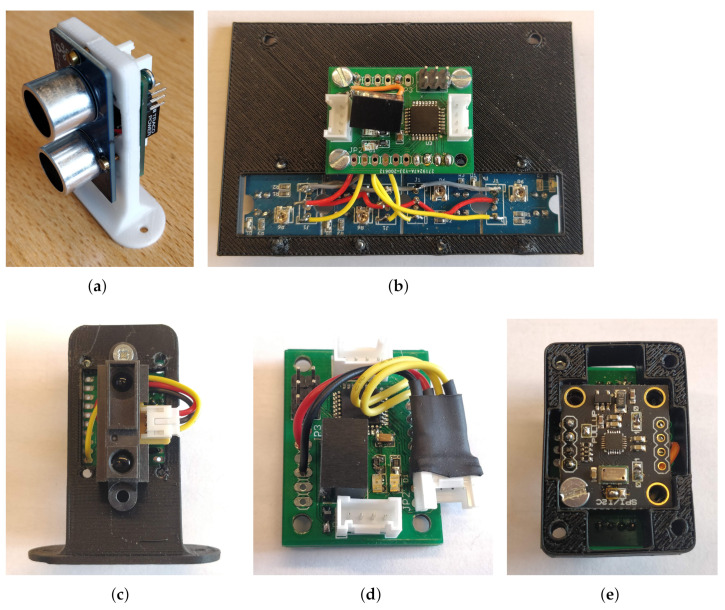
Examples of CAN node for our platform. (a) Ultrasonic distance sensor; (b) Line of ground sensors; (c) Infrared distance sensor; (d) Quadrature encoder node; (e) IMU node.
